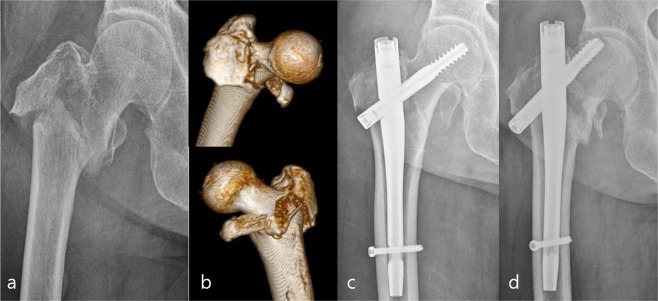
(a) Initial radiograph and (b) 3-D CTs of a 83-year-old woman showing an unstable comminuted pertrochanteric fracture with a basicervical fracture component and detached GT. (c) Postoperative radiograph showing good reduction status. (d) Anteroposterior radiograph showing cut-out of a lag screw 3 months after surgery.
